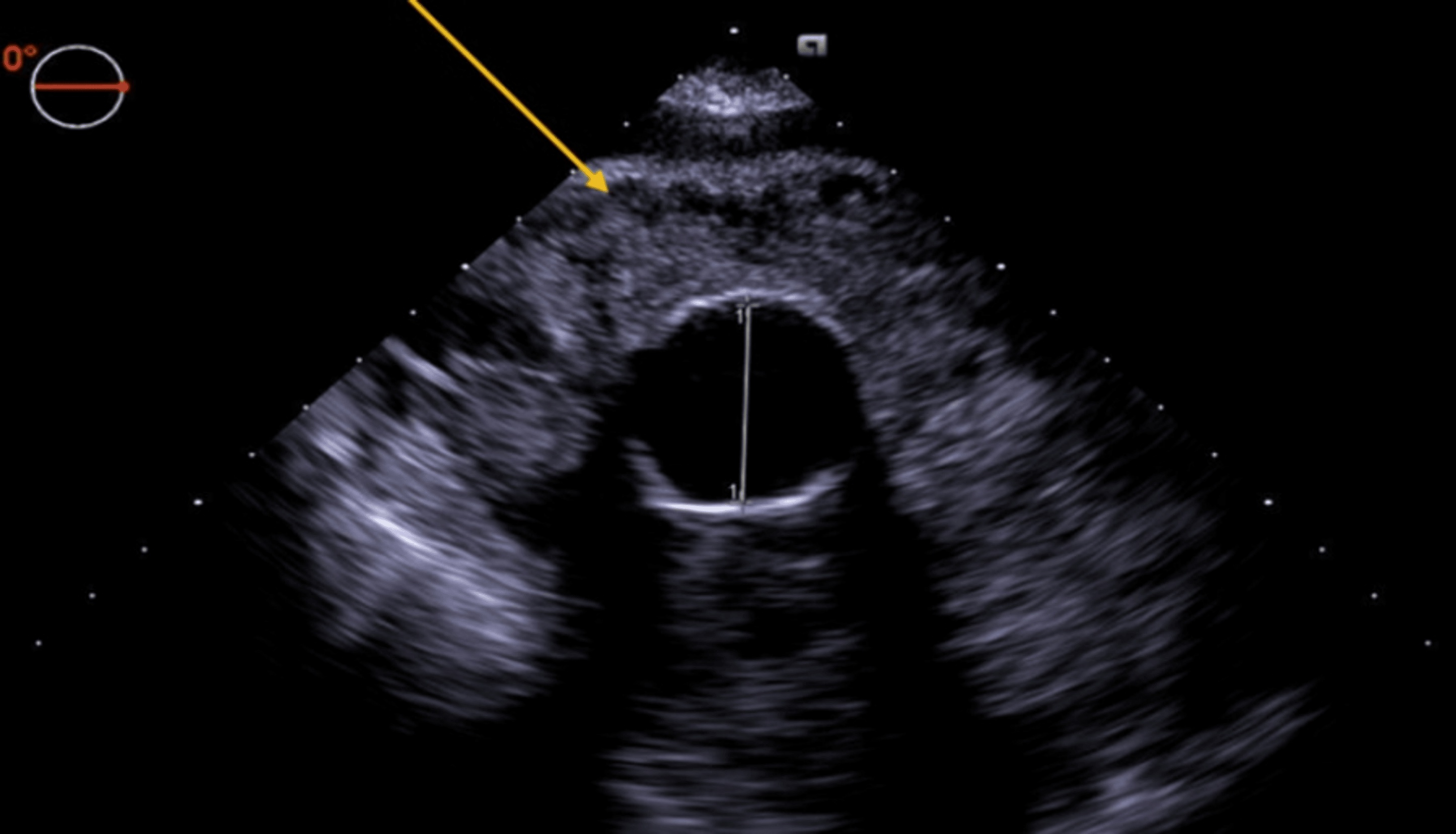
Alternative short-axis transesophageal echocardiogram of the mass.
